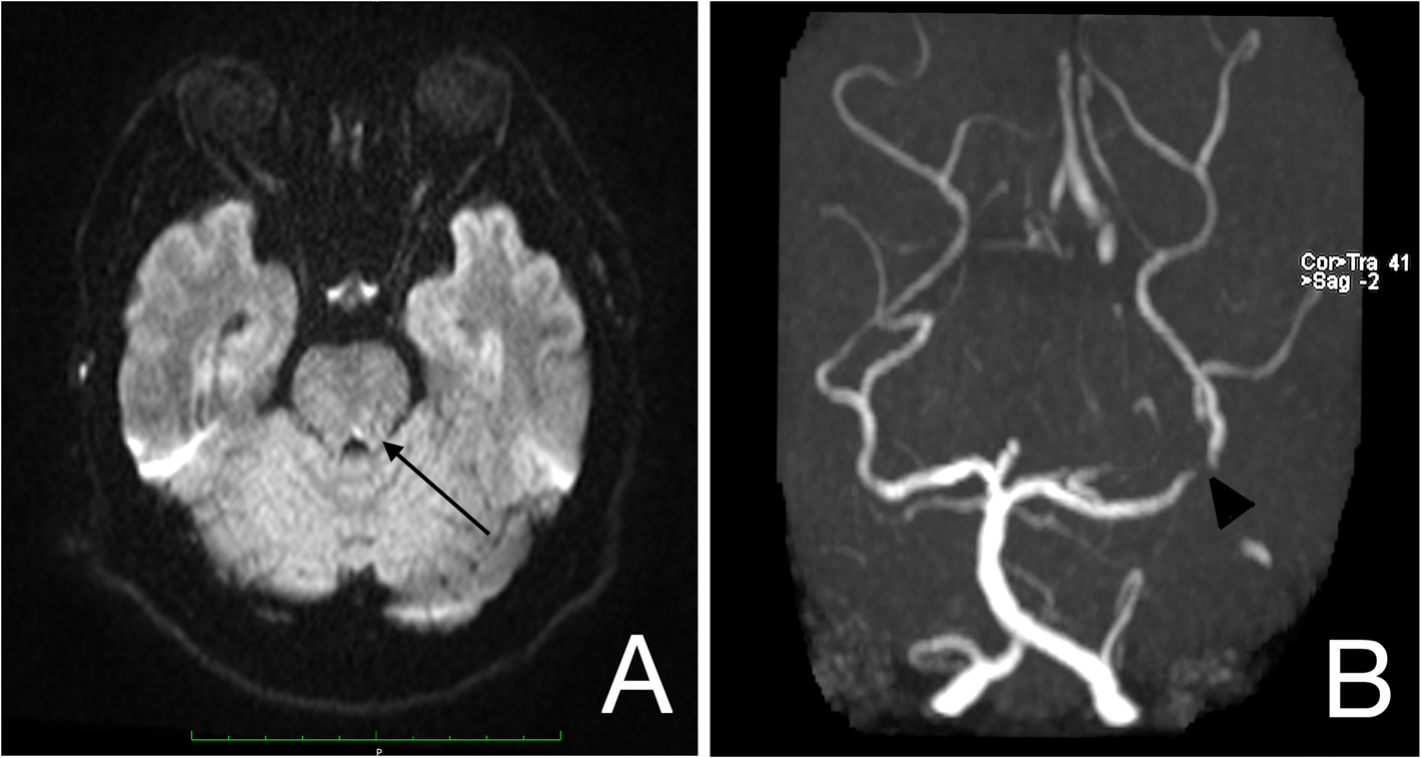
MRI brain of axial diffusion-weighted imaging sequences showed a hyperintense lesion at the left dorsal tegmentum of pontomesencephalic junction (a). MRA revealed narrowing at the P2 segment of the left posterior cerebral artery (b)
